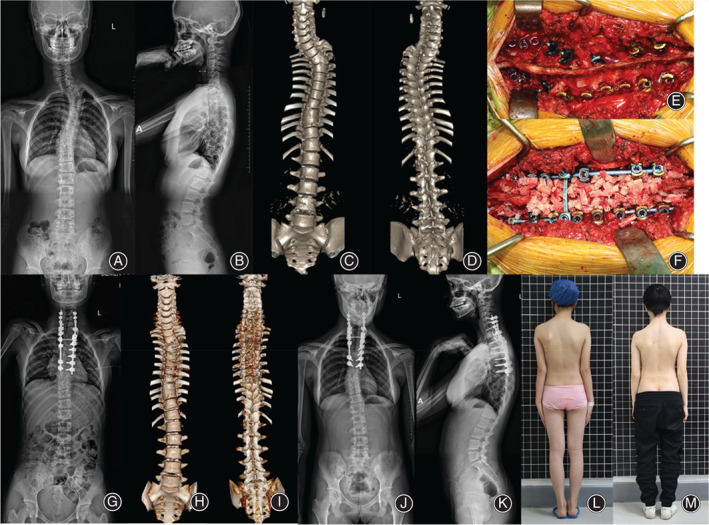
A 13‐year‐old female patient with a T3 hemivertebra underwent posterior correction and fusion surgery without hemivertebra resection. (A, B) Preoperative X‐ray measurement showed a local Cobb angle of 44°,with a T1 tilt angle of 25.3°. (C, D) Preoperative CT showed a T3 hemivertebra with incomplete segmentation. (E, F) A Ponte osteotomy and bone grafting were performed *via* posterior approach surgery. (G) X‐ray immediately postoperatively showed a local Cobb angle of 10.4°, with a correction rate of 76.36%. (H, I) CT showed effective correction without hemivertebra resection. (J, K) Follow‐up X‐ray 3 years after surgery showed satisfactory correction, with no obvious loss of correction. (L, M) The neck tilt improved significantly in appearance after surgery
